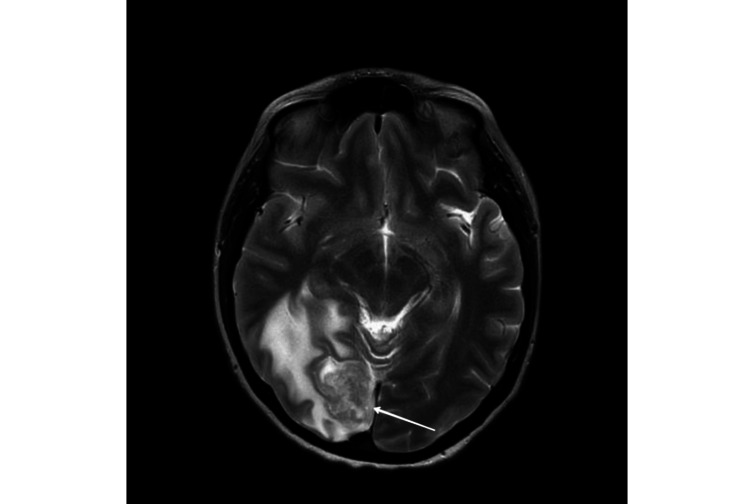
MRI of the head with contrast T2 FLAIR demonstrating occipital metastasis. MRI: magnetic resonance imaging; FLAIR: fluid-attenuated inversion recovery
